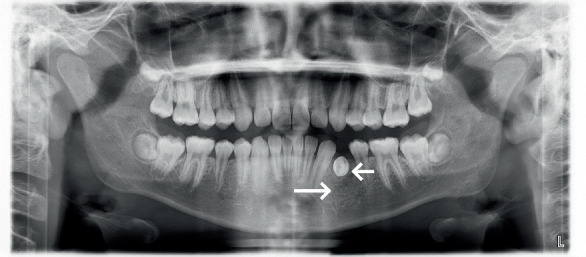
Panoramic radiograph: 2 months after operation. The panoramic radiograph showed that the transmission shadow of the apical area disappeared, bone mineral density increased (long arrow), and the buccal–lingual impacted image in Tooth 34 (short arrow).
